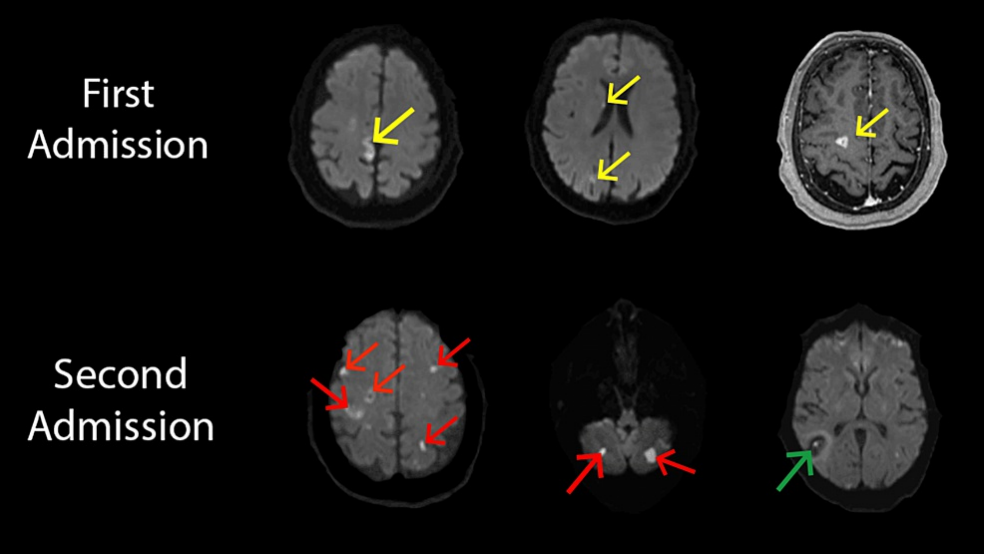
Multifocal ischemic strokes (yellow arrows) on magnetic resonance imaging (MRI) brain obtained during the first admission compared to new multifocal ischemic strokes (red arrows) as well as hemorrhagic conversion of the old parietal infarct (green arrow) on MRI from the second admission
